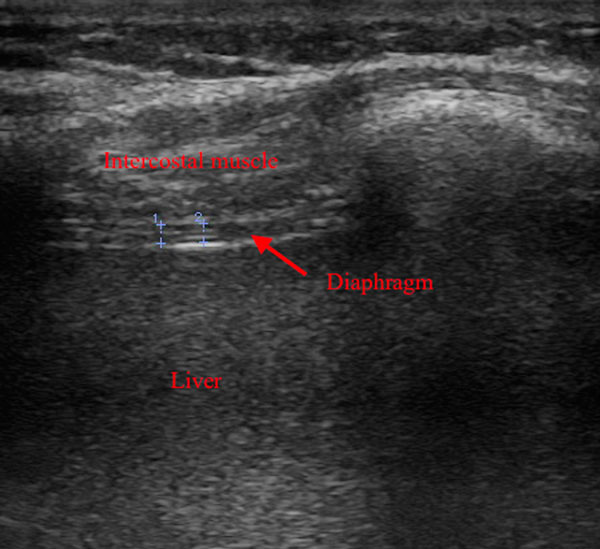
Sample ultrasound recording. Lines 1 and 2 are diaphragm thickness measurements.
